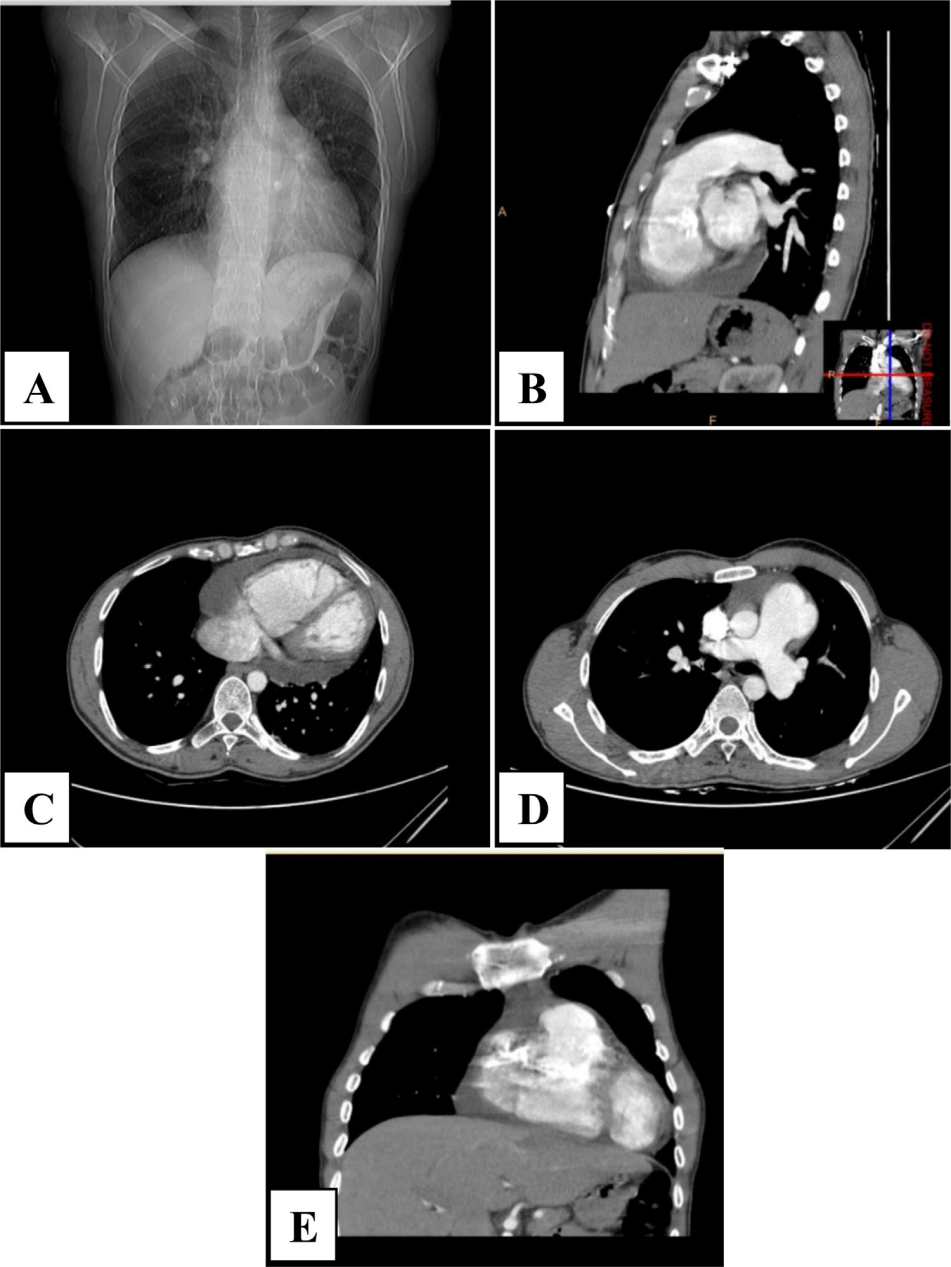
Pulmonary CT angiography: A, scout image; B, sagittal view; C, axial view; D, axial view; E, coronal view. Arrows in panels B, D, and E highlight the focal aneurysmal dilation of the right ventricular outflow tract.
